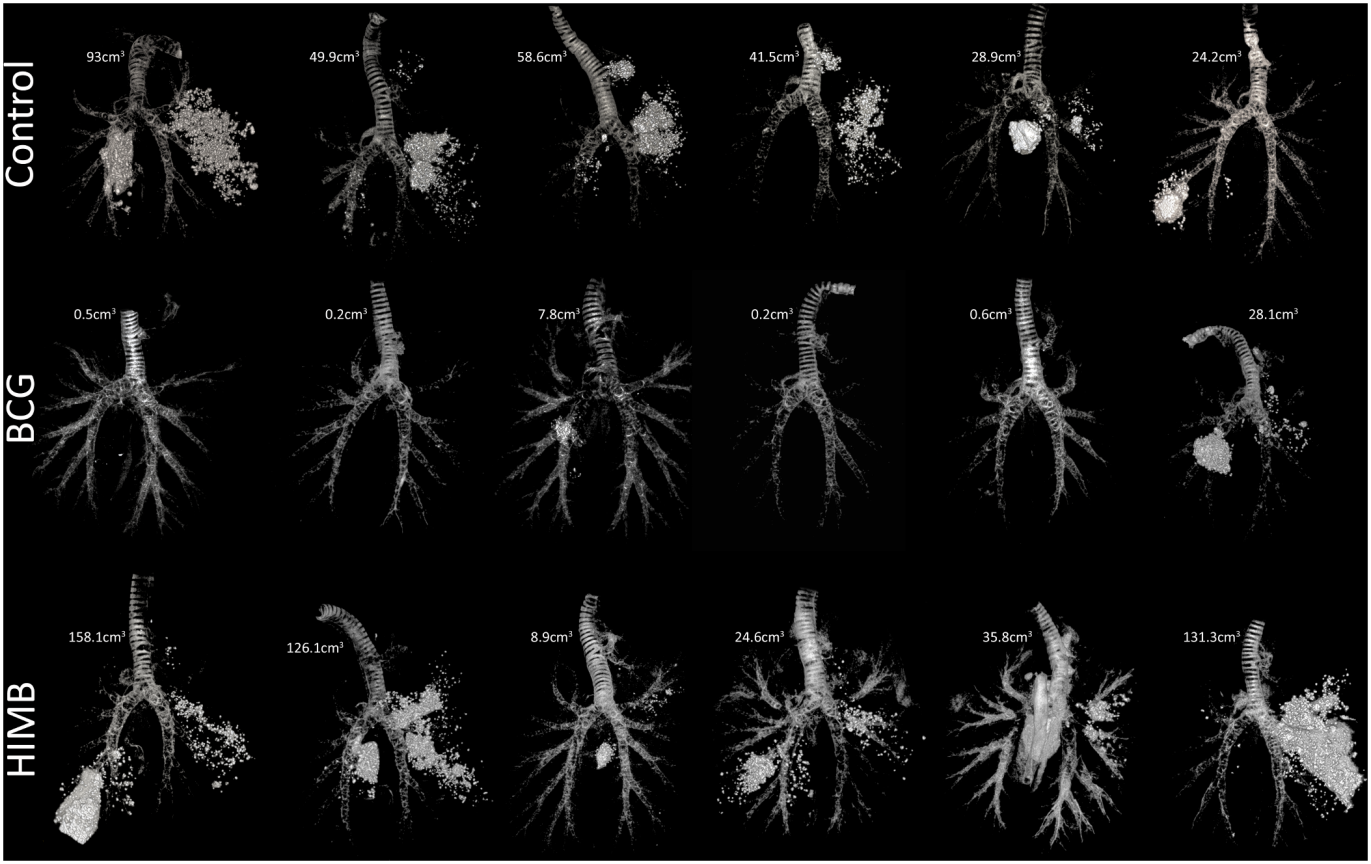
Individual volume of pulmonary gross lesions measured by computed tomography (CT). The figure shows rendering images of the total lesion volume (expressed in cm^3^) for each lung of the 6 unvaccinated control, 6 BCG vaccinated and 6 HIMB vaccinated lambs. The trachea and the bronchial tree are also displayed for anatomical reference.
